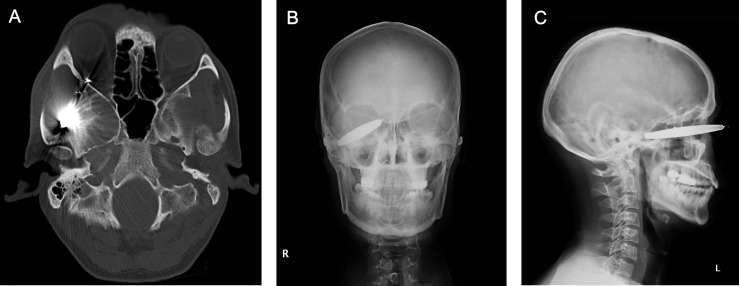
Orbital imaging: (A) axial CT scan, (B) anteroposterior X-ray and (C) lateral X-ray, all showing penetration of the foreign body through the orbital cavity into the inferior orbital fissure, with the globe itself remaining intact.
